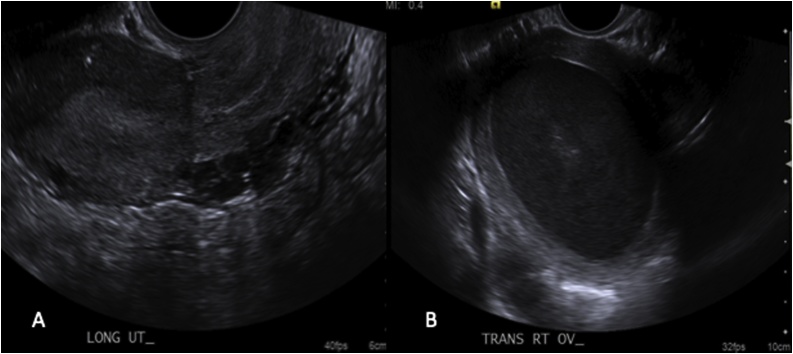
2D transvaginal Pelvic ultrasonography: A. Left unicornuate uterus, B. Hematometra in the non-communicating rudimentary horn was reported as right ovarian endometrioma.
